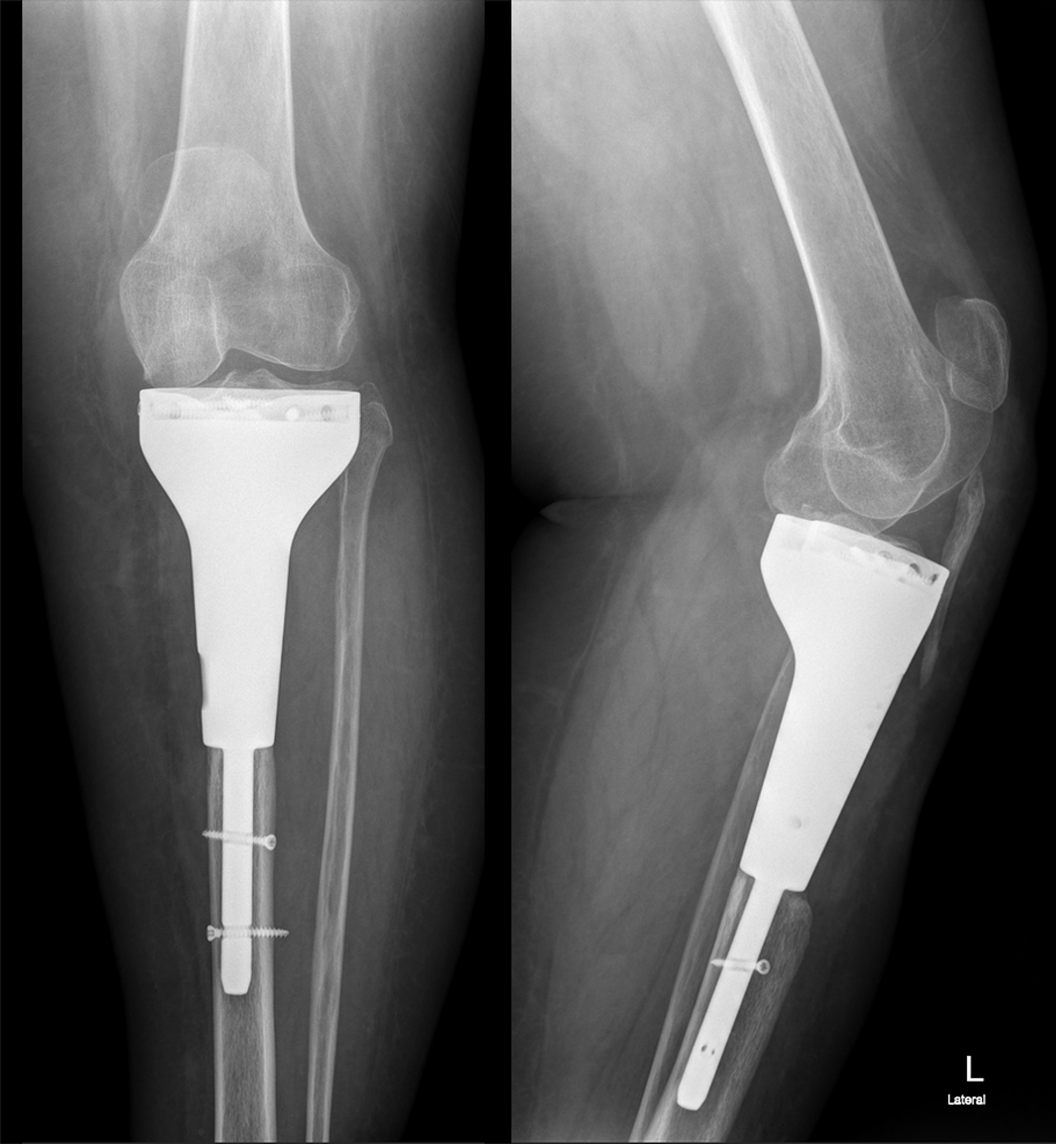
Postoperative first-year X-rays
